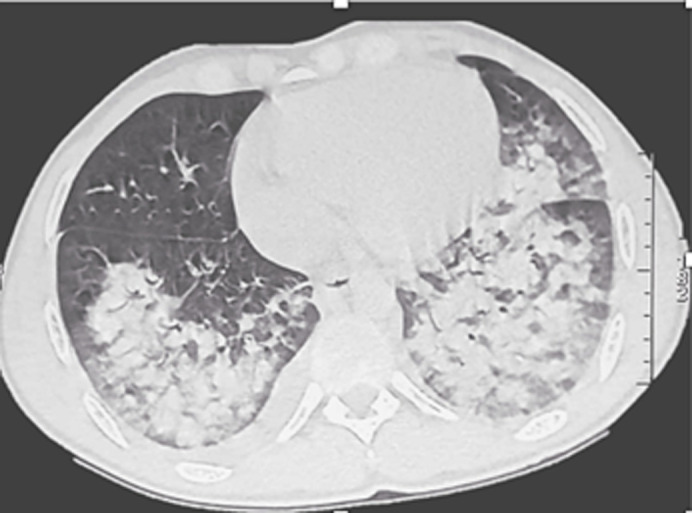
thoracic computed tomography showing bilateral parenchymal condensations with predominantly central ground glass opacities and bilateral pleural effusion
